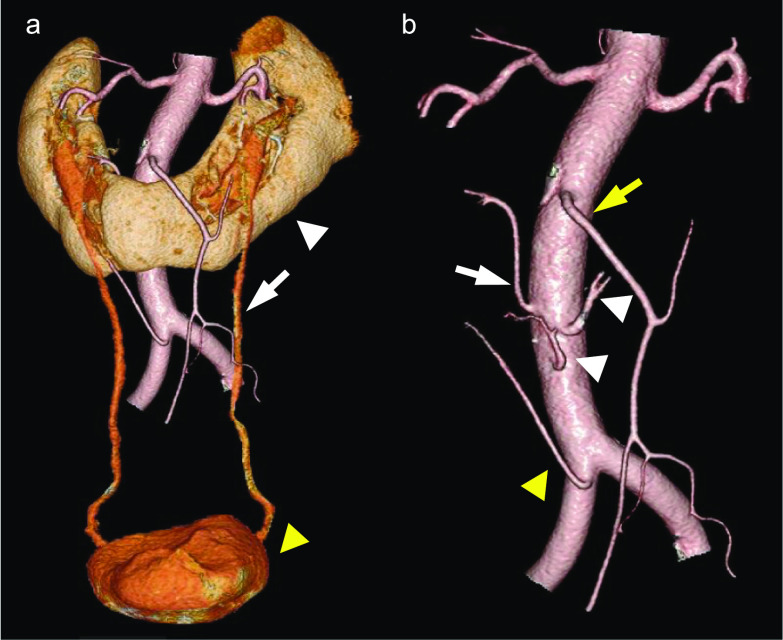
Three-dimensional computed tomography imaging showing the horseshoe kidney and aberrant renal arteries. a The horseshoe kidney (white arrowhead), left ureter (white arrow), and bladder (yellow arrowhead). b The root of the aberrant renal arteries at the isthmus from the aorta (white arrowhead), right branch of the aberrant renal artery from the aorta (white arrow), right branch of the aberrant renal artery from the right common iliac artery (yellow arrowhead), and inferior mesenteric artery (yellow arrow)
